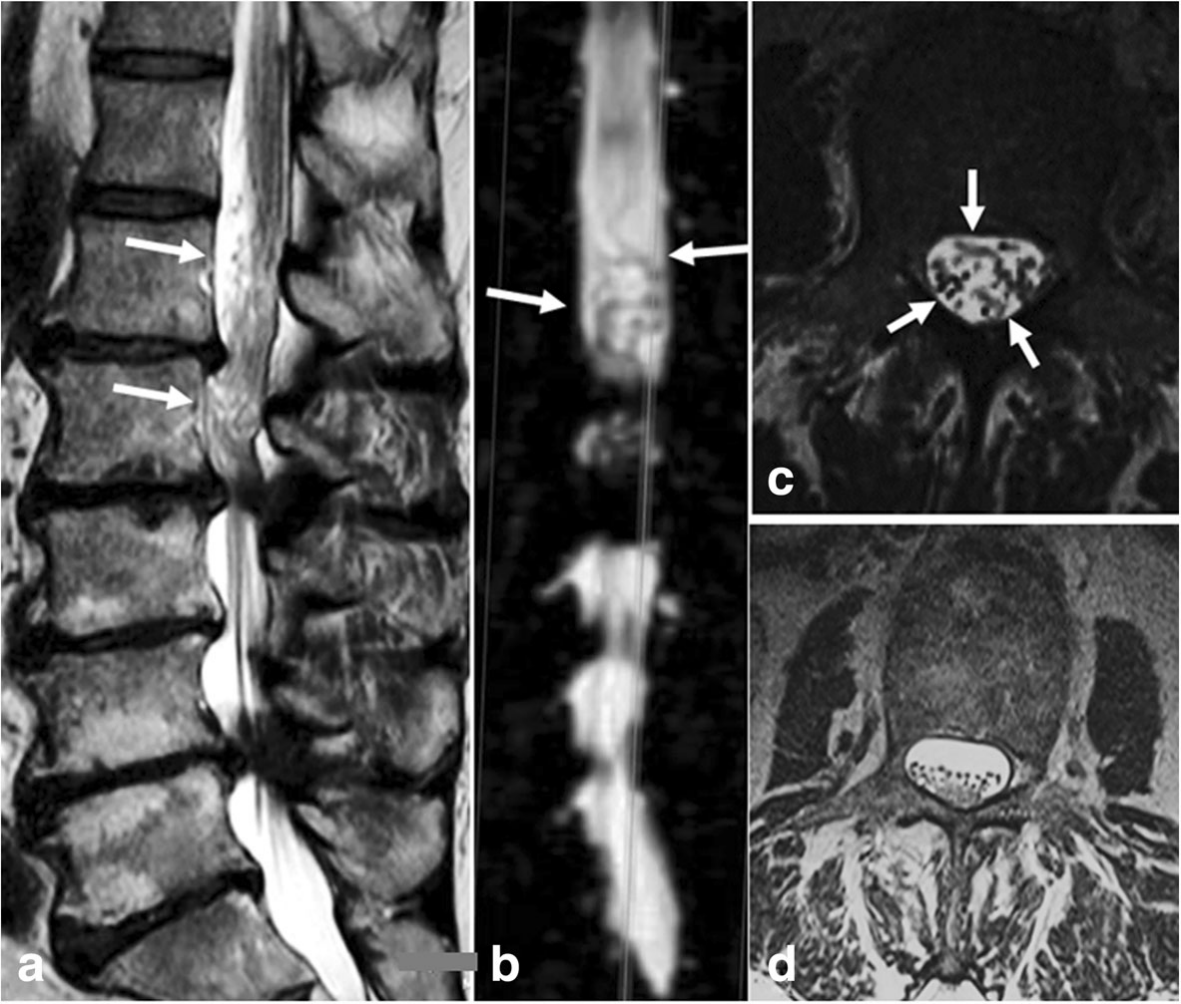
Sagittal T2-WI of spinal canal stenosis with evidence of loop-shaped RNR a) Sagittal T2-WI of spinal canal stenosis L2/L3. At this level caudal straightened nerve roots switch to loop like RNR (white arrows); b) The coronal Myelo-MRI shows the horizontalized loops (white arrows); c) Axial T2-WI shows segments of the loops (white arrows); d) Axial T2-WI of a normal lumbar spinal canal: the cauda roots appear as dots
